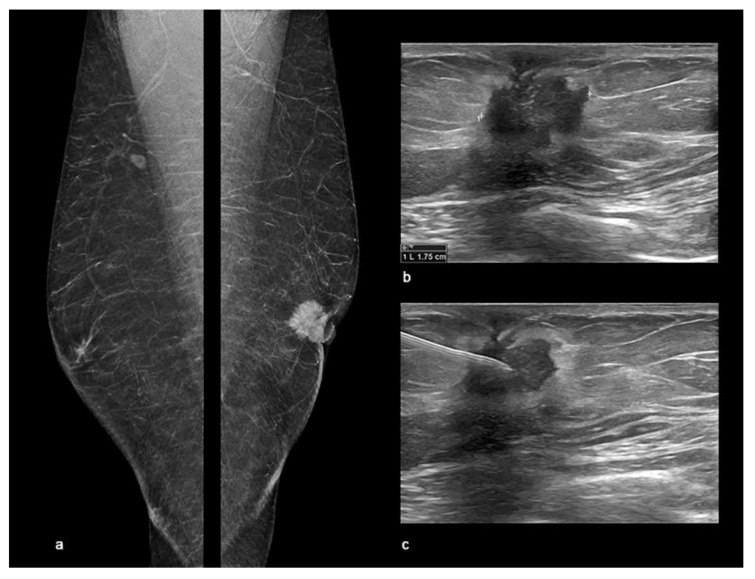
Male Breast Cancer. Digital breast tomosynthesis (DBT) and ultrasound (US) showing an invasive ductal carcinoma of the left breast in a 58-year-old male observed at our department with a palpable retro-areolar mass and nipple retraction at clinical examination. (a) The synthetic 2D reconstructed medio-lateral-oblique images (s2D-MLO) showed a sub-areolar dense mass with spiculated margins and skin retraction. (b) US showed a left retro-areolar, hypoechoic mass with indistinct border and spiculated margins of 1.75 cm. (c) US-guided Fine-Needle Aspiration Cytology (FNAC) resulting C5 according to the the IAC Yokohama System for Reporting Breast Fine-Needle Aspiration Biopsy Cytopathology (1st Edition, 2020).
